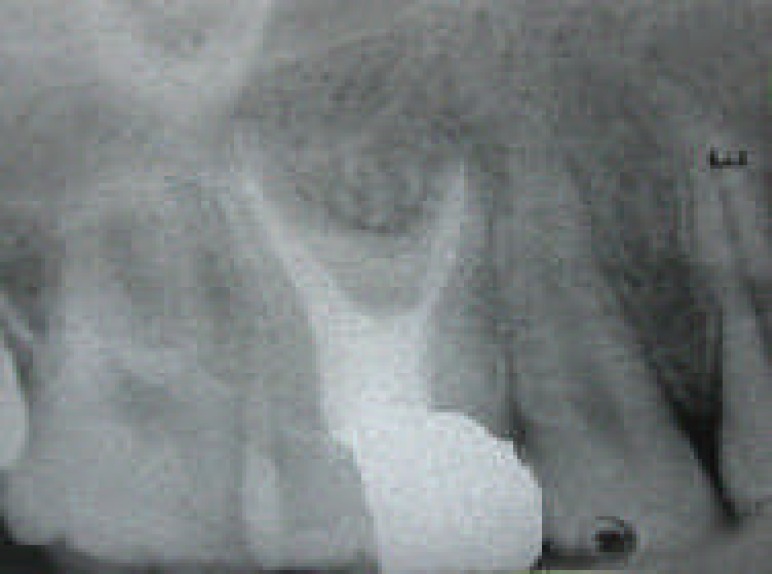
Radiographic image of the tooth one year after a complete root canal therapy
